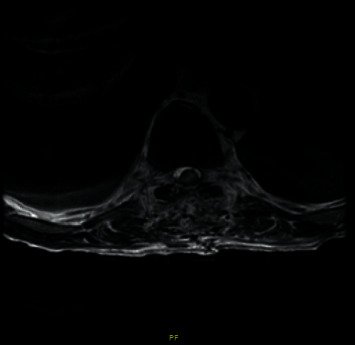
Postoperative MRI axial view showing the little fluid collected at the intradural and extramedullary space where the tumor was located.
